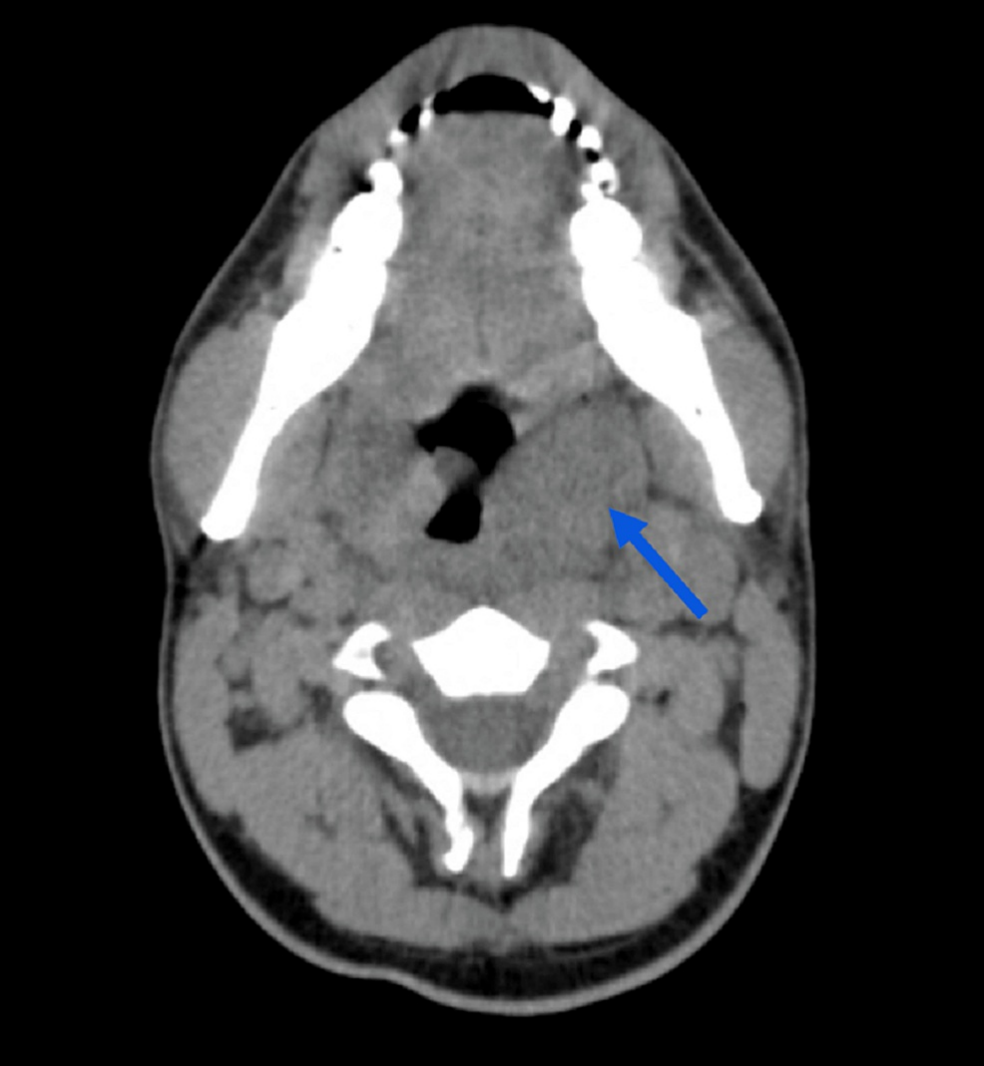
Axial computed tomography angiogram of the neck vessels showing heterogeneous and asymmetric hypertrophy of the left tonsillar pillar (blue arrow)
